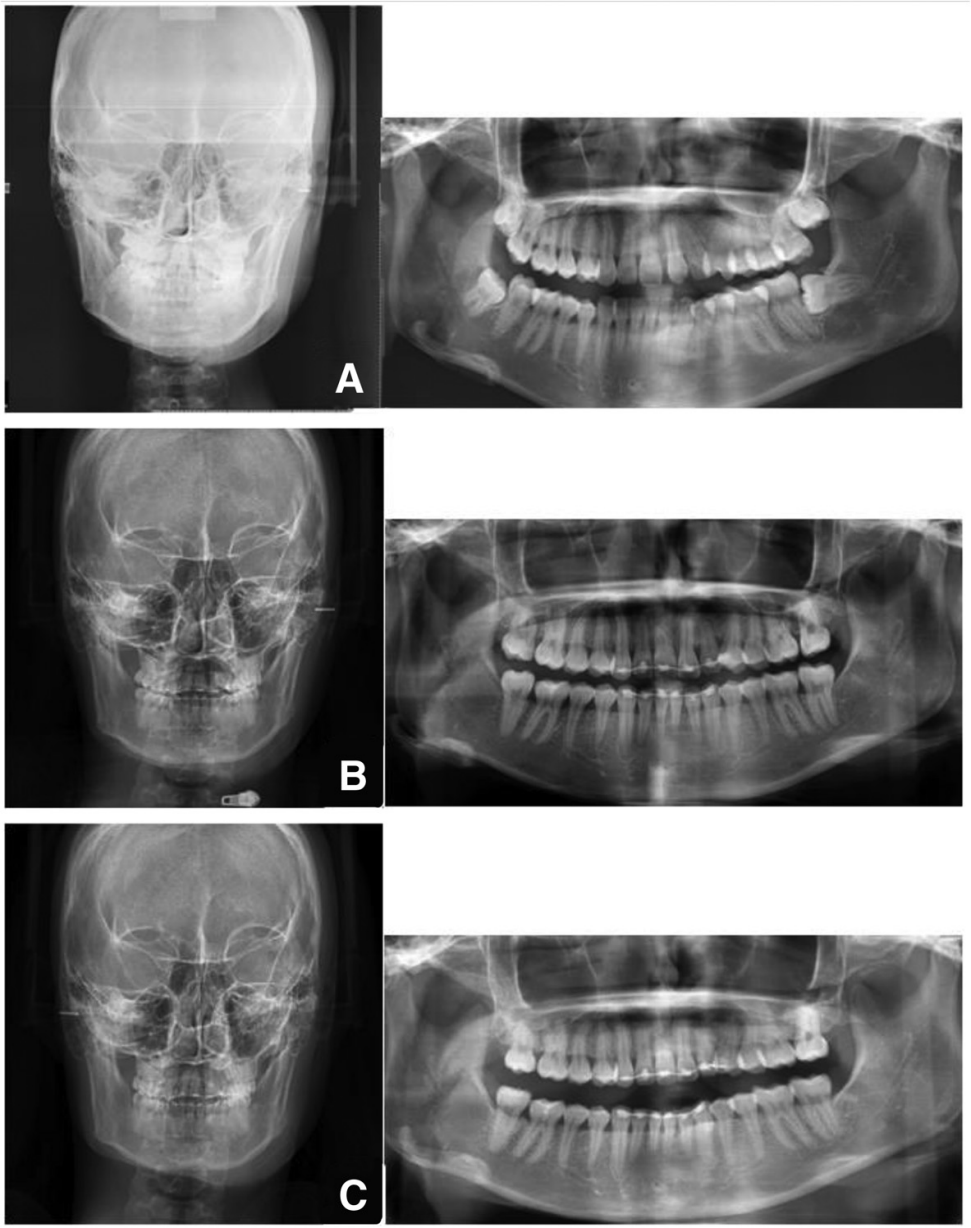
Radiographies were taken on a 2 July 2010, b 1 December 2012, and c 13 December 2014
